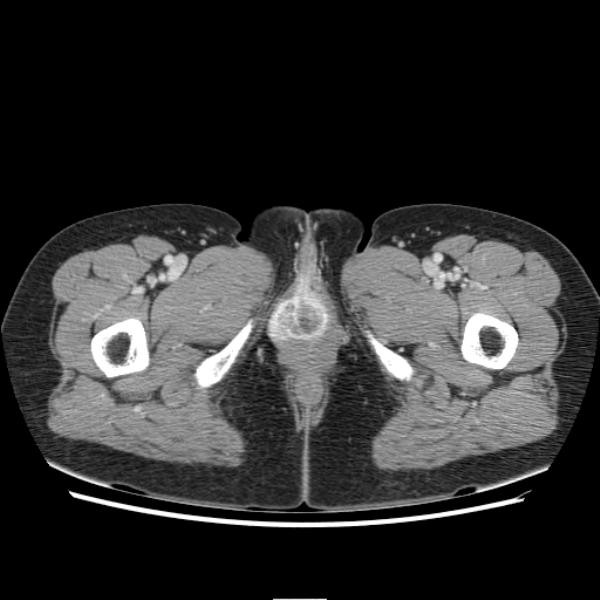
Enhanced pelvic CT scan demonstrates a heterogeneously enhanced mass on female urethra.
